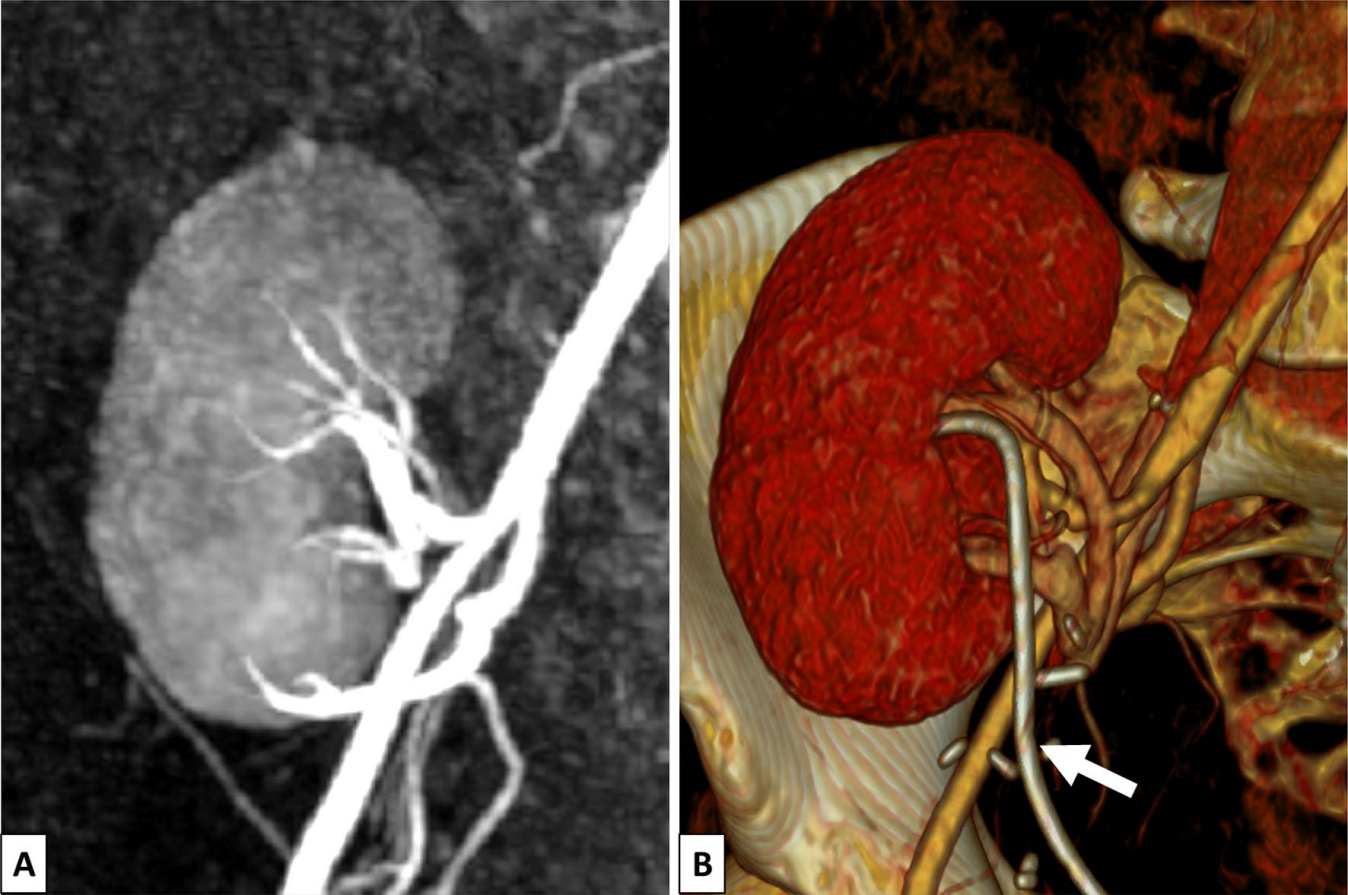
MR angiography (A) and CT angiography (B) with 3D reconstruction of the transplant kidney. The fistula could not be detected in either examination. At the time of the CT angiography, a double J-stent was implanted (white arrow)
